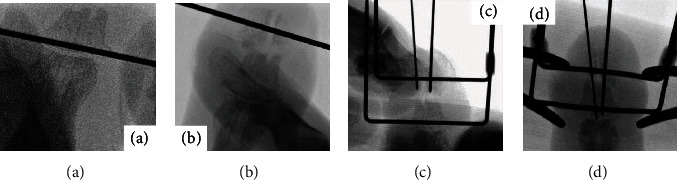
Intraoperative X-ray. (a) A 1.2 mm fixator pin was inserted from the base of the distal phalangeal bone transversally. (b) A 1.2-mm fixator pin was inserted from the distal one-third of the distal phalangeal bone transversally. (c) The parallel ends of the two pins were inserted. (d) Compression of fracture with Kocher.
